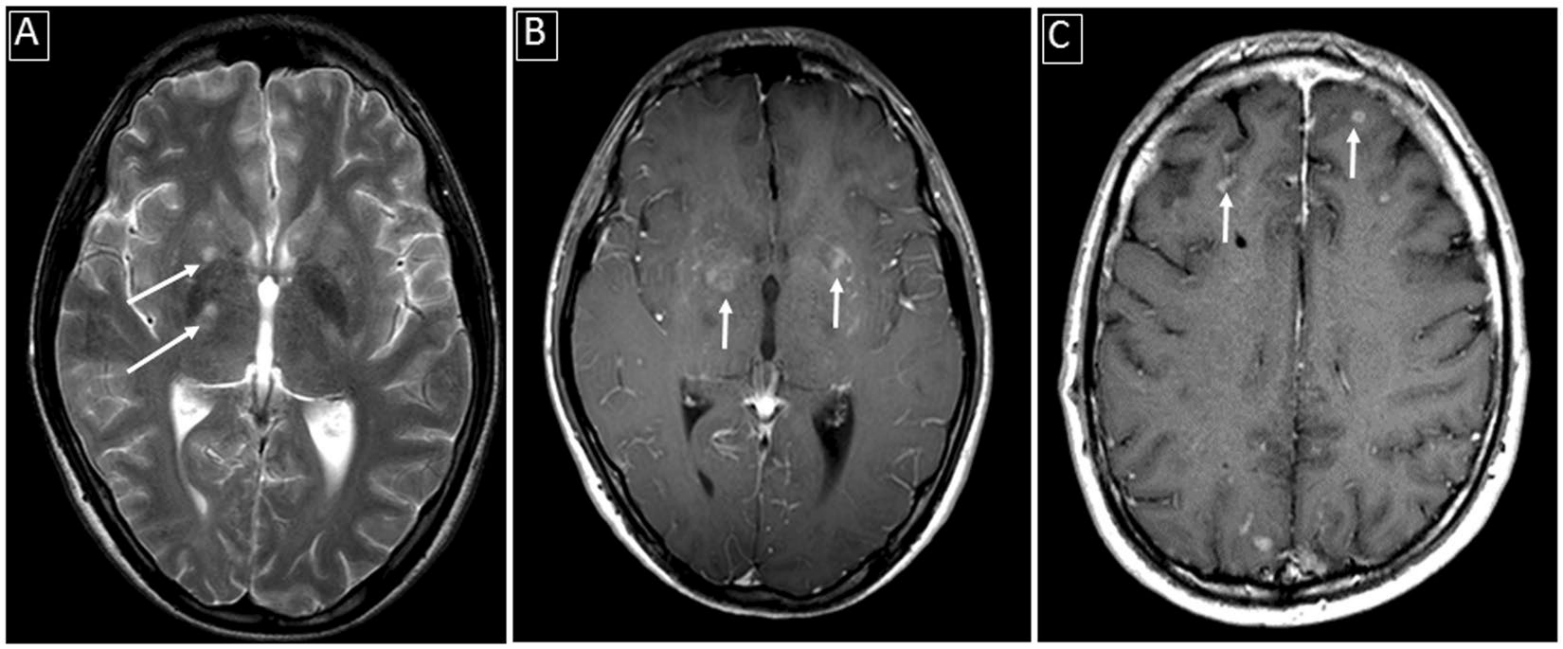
Examples of parenchymal involvement in CNS cryptococcosis: (A) Abnormally enlarged perivascular spaces in the basal ganglia bilaterally (white arrows) on T2-weighted image; (B) Abnormal enhancing lesions in the basal ganglia (white arrows) in the same patient as A on enhanced T1-weighted image; (C) Superficial focal parenchymal enhancing lesions (white arrows) adjacent to sulcal meningeal enhancement.
